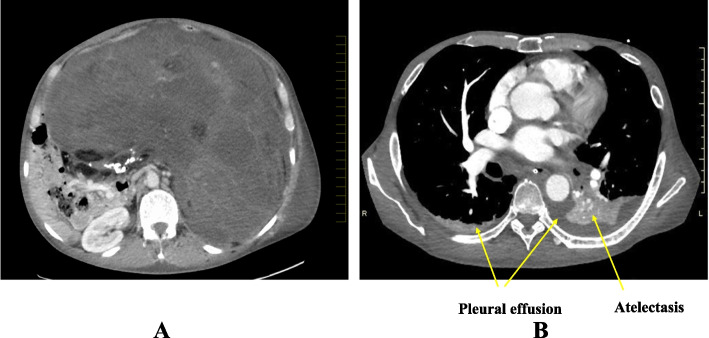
The patient’s CT images on admission to the intensive care unit (ICU). A giant retroperitoneal tumor occupied his abdominal and pelvic region, and severe compression of the inferior vena cava (A), bilateral pleural effusion, and atelectasis (B) were observed
